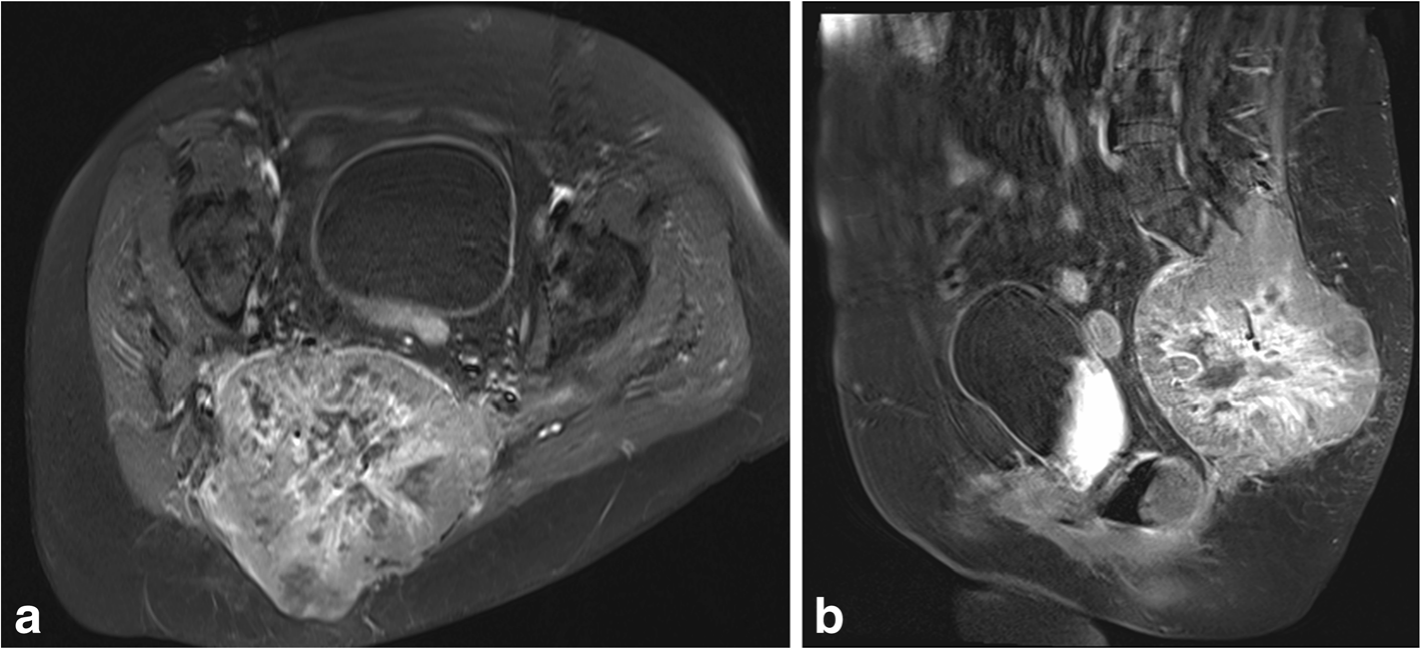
Baseline postcontrast T1-weighted magnetic resonance images (a: axial plane; b: sagittal plane). Preinterventional images depicting the large (11.9 × 11.6 × 14.9 cm) viable tumor mass with infiltration and destruction of the os sacrum as well as infiltration of the sacral nerve plexus.
